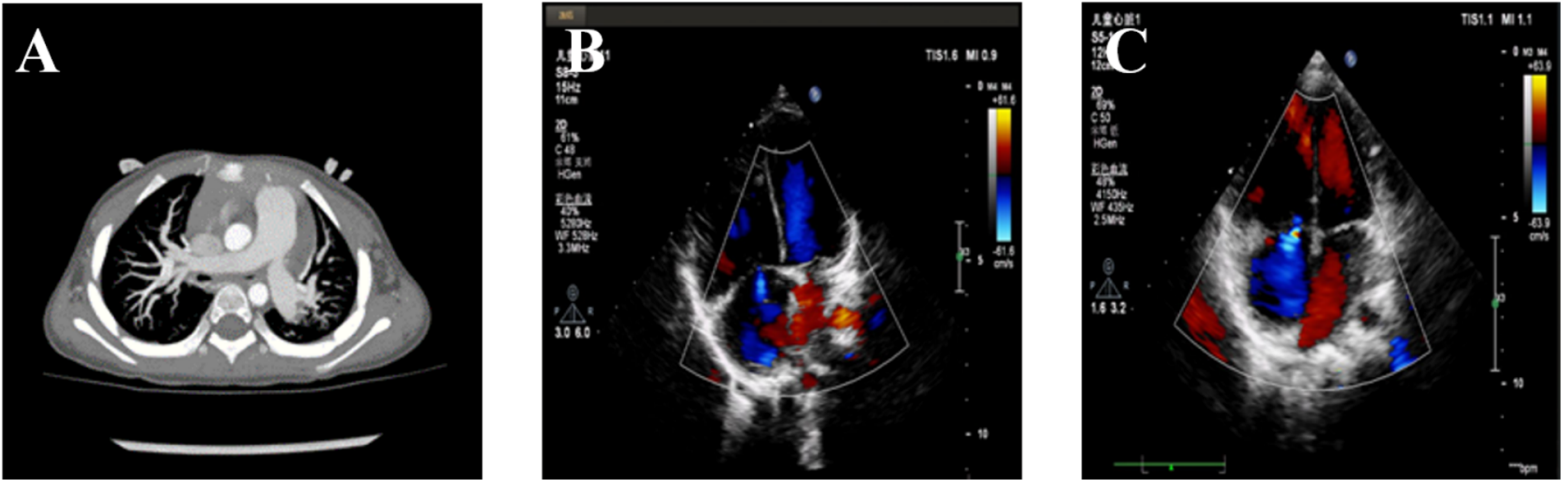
Pulmonary arterial hypertension of the patient. (A) The computerized tomography scan shows a dilated pulmonary artery. (B) The echocardiogram shows tricuspid regurgitation prior to treatment. (C) The echocardiogram shows tricuspid regurgitation after treatment.
